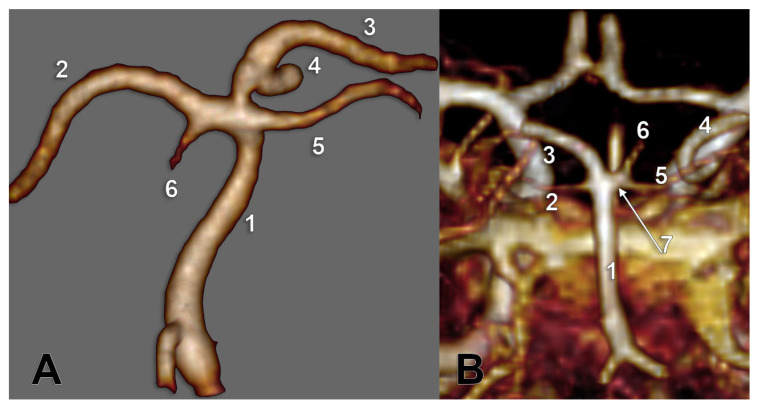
(A) Posterior view of the basilar artery, with a computed tomography angiogram, and three-dimensional volume rendering. The right posterior cerebral artery appears as a superior median branch of a trifurcated basilar artery. 1. BA; 2. left PCA; 3. right PCA; 4. posterior communicating artery; 5. right SCA; 6. left SCA. (B) Common trunk of origin of the superior cerebellar artery and the artery of Percheron. MR angiogram, three-dimensional rendering. Posterior view. 1. BA; 2. left SCA; 3. left PCA; 4. fPCA; 5. right SCA; 6. aP; 7. common trunk of right SCA and aP.
